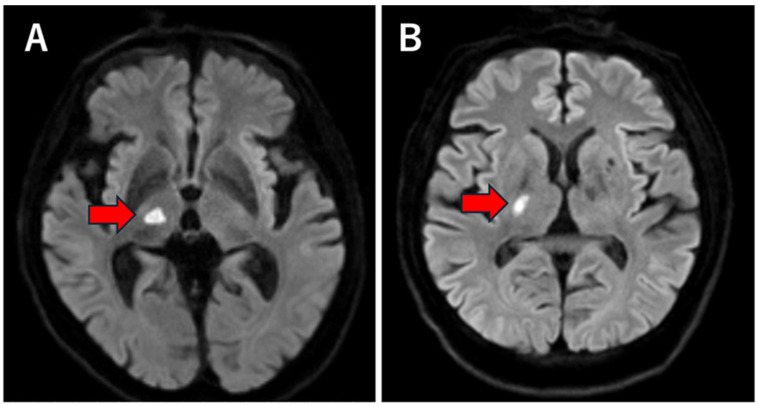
Representative axial diffusion-weighted imaging (DWI) of perforator infarction. (A) Axial DWI demonstrating a small, unilateral acute infarction in the LSA territory involving the internal capsule/basal ganglia (arrow). (B) Axial DWI demonstrating a unilateral acute thalamic infarction confined to the thalamus without cortical involvement (arrow). DWI, diffusion-weighted imaging; LSA, lenticulostriate artery.
